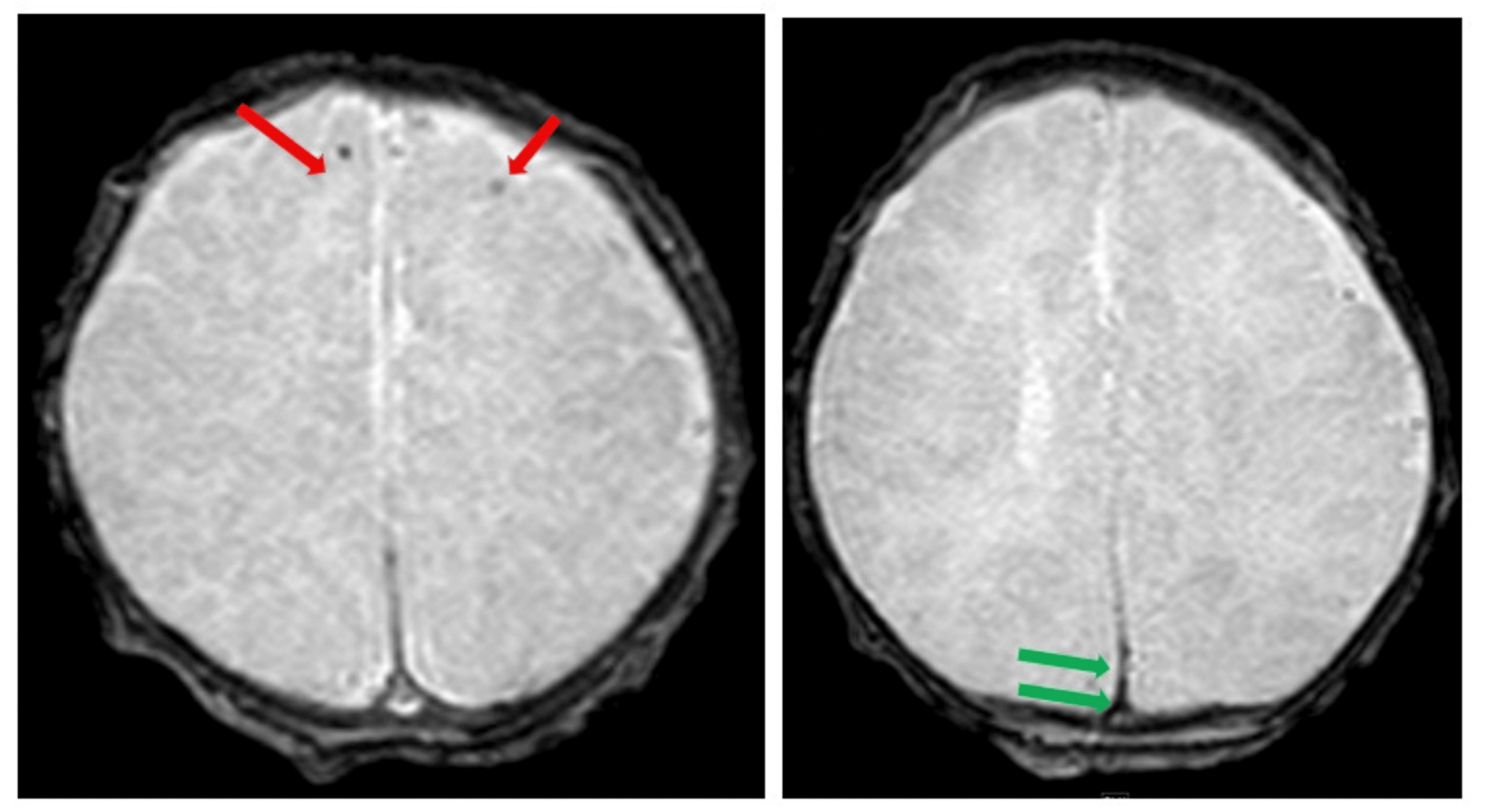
Brain MRI shows few punctate foci of susceptibility-related signal loss along the surface of the bilateral frontal lobes (left; red arrows), along the posterior falx cerebra (right; green arrows), and bilateral tentorial leaflets
